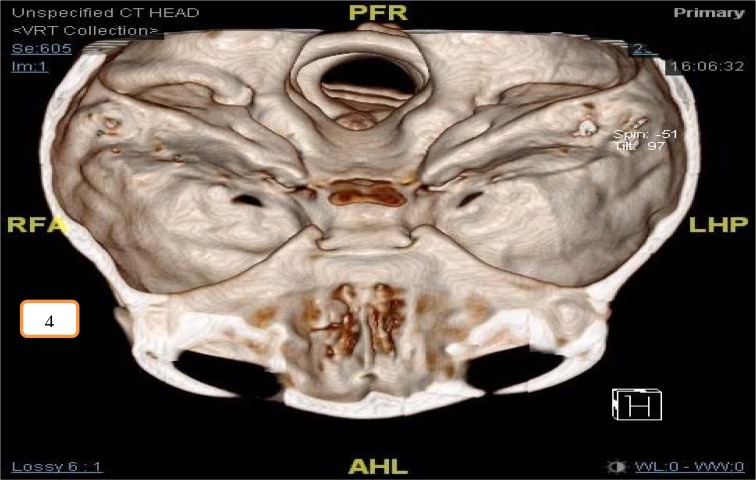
Three-dimensional (3D) images reconstructed from the fine cut CT scan of the brain, skull base and paranasal sinuses showed right cribriform plate fracture.
